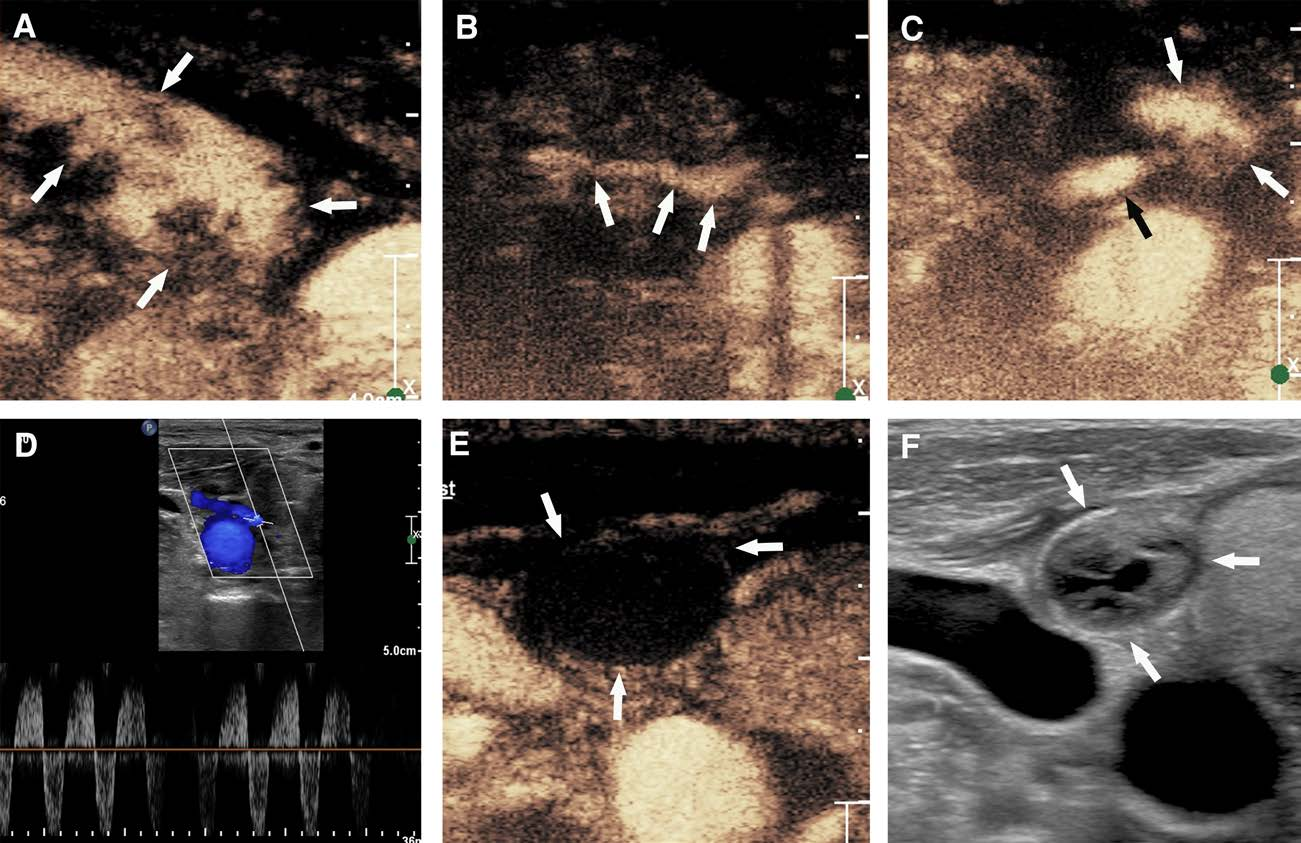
US Scans of the bleeding before, during, and after Radiofrequency Ablation. CEUS showed that Acute hemorrhage of right neck (Panel A, white arrow), the source of bleeding is the inferior thyroid artery (Panel B, arrow). After ablation, the enhancement range was significantly reduced, Pseudoaneurysm formation (Panel C, white arrow),the neck of the pseudoaneurysm (Panel C, black arrow).Spectral Doppler revealed a typical to-and-fro flow (bidirectional) (Panel D).Thirty-six hours later, CEUS showed that a thrombus filled the whole cavity (Panel E, white arrow). US showed that the hematoma was smaller (Panel F, white arrow). CEUS = contrast-enhanced ultrasound, US = ultrasonography.
